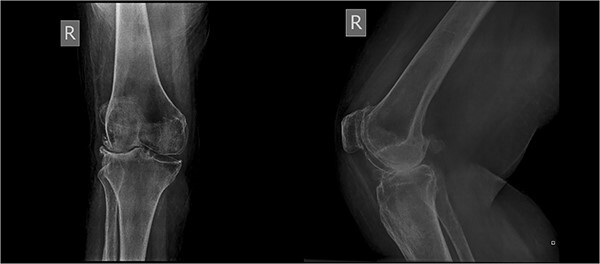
Preoperative X-rays demonstrating stage IV OA.
